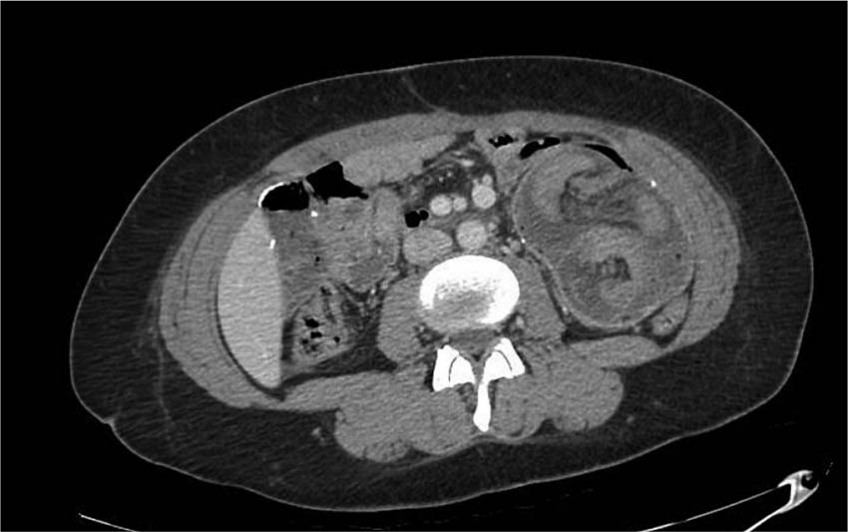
CT of the jejunojejunostomy intussusception.
